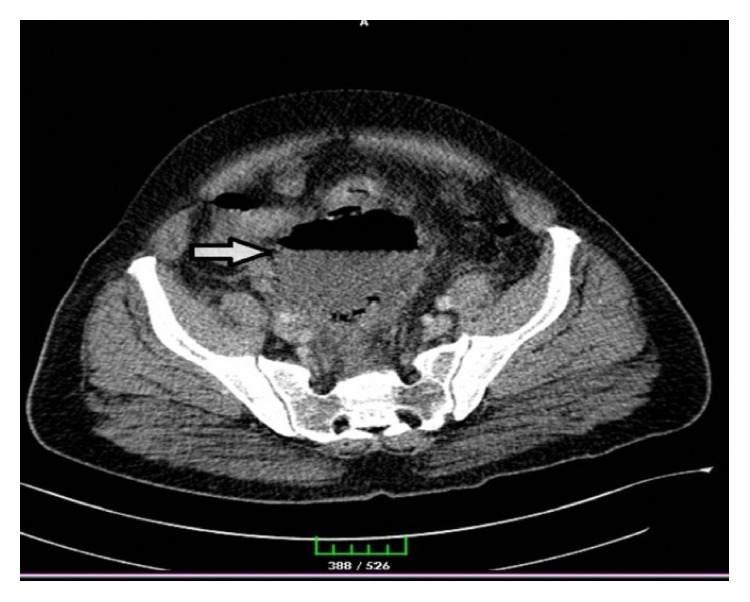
CT image showing 98 × 95 mm abscess with air-fluid levels at the entrance to the pelvic cavity.
